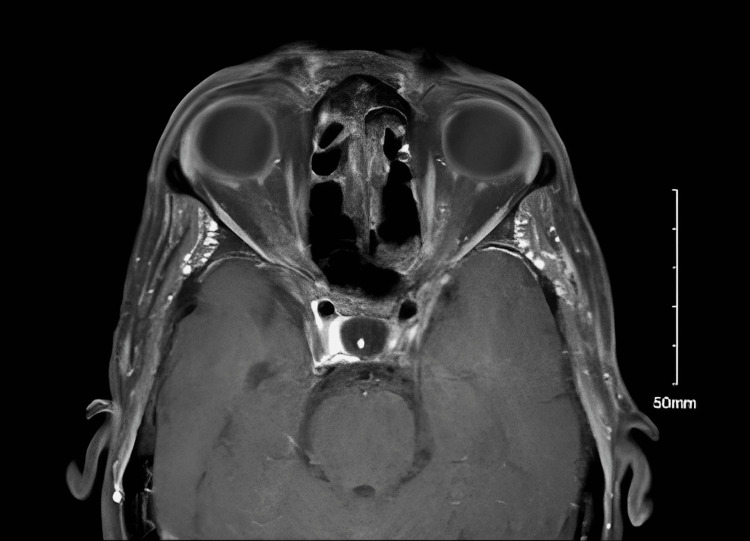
Orbital MRI with contrast, cross-sectional view This slide shows left optic nerve peripheral enhancement with adjacent fat stranding. Similar findings were found in the right optic nerve in the other slides (pictures not shown)
